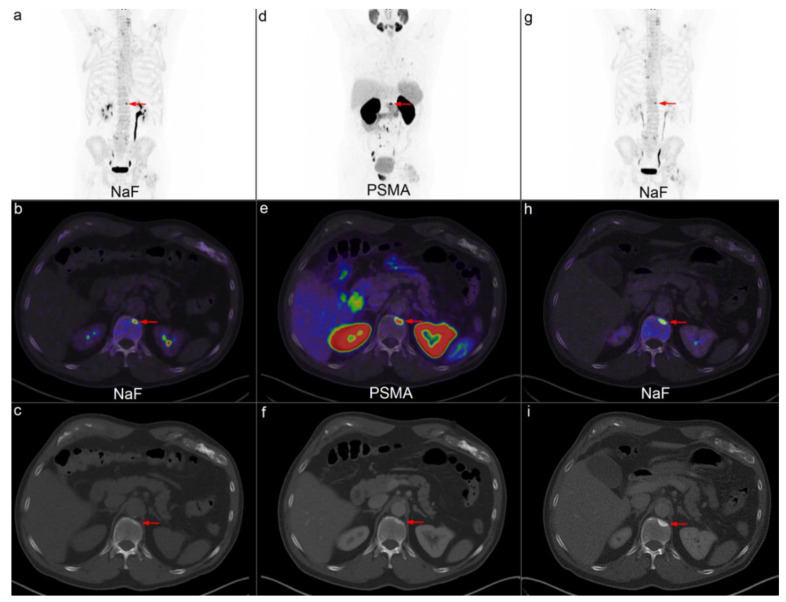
Example of a patient referred for ^18^F-NaF PET/low-dose CT (ldCT) for primary staging. An inconclusive lesion (*red arrow*) with increased uptake on ^18^F-NaF PET/ldCT is noticed in Th12 on maximum intensity projection (MIP) (a) and in fused axial projection (b). No correlating lesion is observed on ldCT (c). Subsequent ^68^Ga-PSMA PET/CT showed increased uptake (d,e). Nevertheless, there was no visible lesion on CT (f). Five months later, an additional ^18^F-NaF PET/CT scan showed increased uptake (g,h). A sclerotic lesion was visible on CT by that time (i). Several lymph node metastases and lung metastases are noticed on the PSMA MIP (d).
